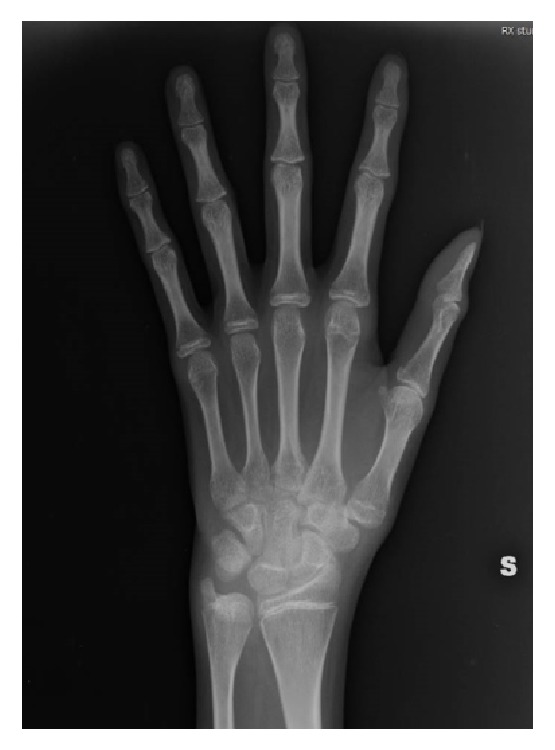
Left hand and wrist X-ray. The ossification of the epiphysial cartilage of distal end of radius and ulna, 1st metacarpus, and phalanxes is not yet complete.
